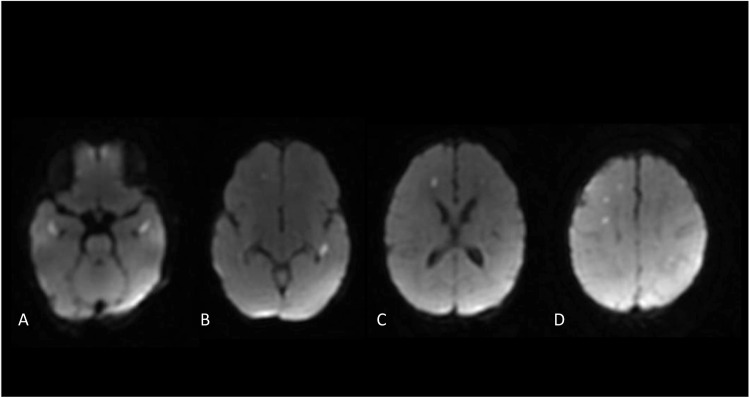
DWI images at different brain levels (pons, mesencephalon, basal ganglia, and corona radiata) of the same patient demonstrate multiple foci of restricted diffusion, confirmed on ADC maps, involving the periventricular and subcortical white matter and distributed along the course of deep medullary veins
